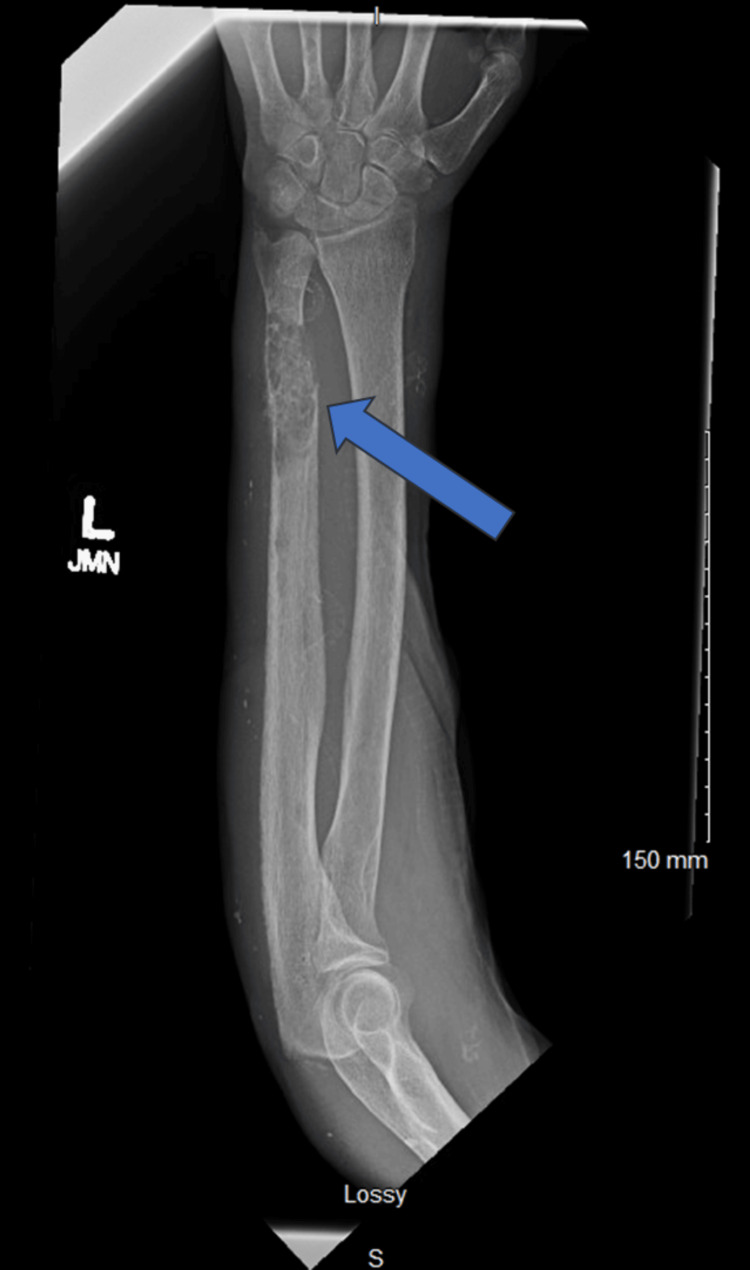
X-ray showing left distal ulnar lytic lesion.
